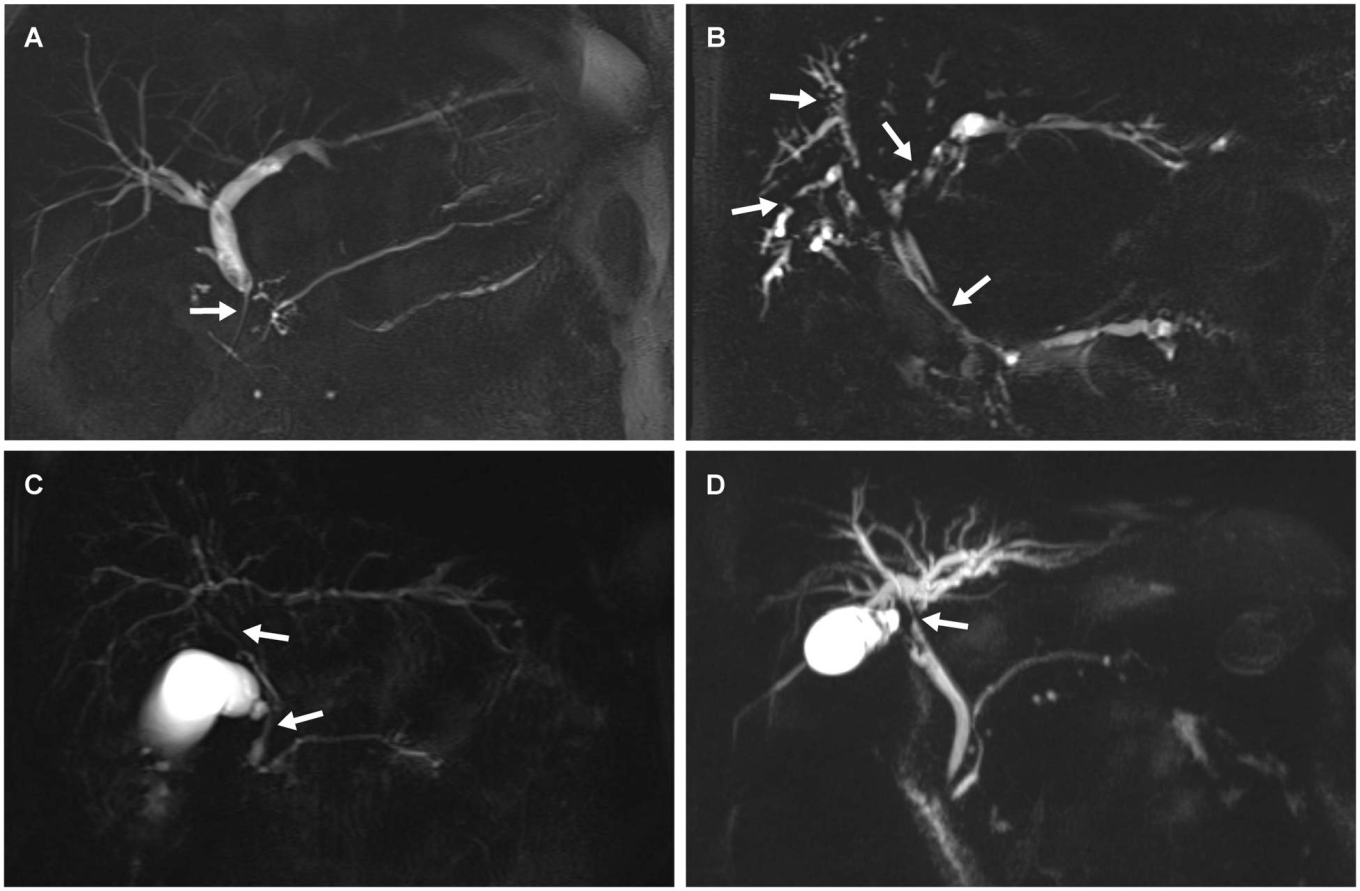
Exemplary MRI/MRCP images of patients with different types of IRC. Affected bile duct areas are marked with arrows. (A) A 52-year-old male patient with an inflammatory stricture of the distal common bile duct (IRC type 1). (B) A 52-year-old male patient with an inflammatory distal stricture of the common bile duct and multiple strictures in the intrahepatic bile ducts with prestenotic dilatations (IRC type 2a). (C) A 59-year-old male patient with a hilar and distal inflammatory stenosis of the common bile duct (IRC type 3). (D) A 45-year-old male patient with an isolated hilar inflammatory stenosis (IRC Type 4). IRC = IgG4-related cholangitis, MRI/MRCP = magnetic resonance imaging/cholangiopancreatography.
